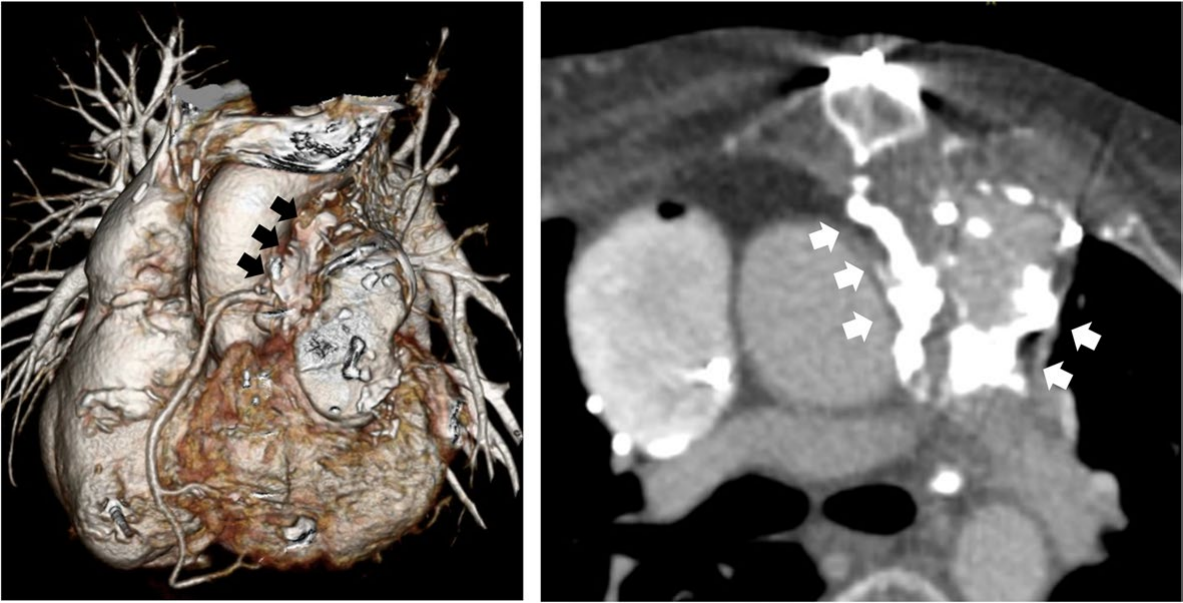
Preoperative computed tomography. The black and white arrows indicate severe calcification around the conduit
